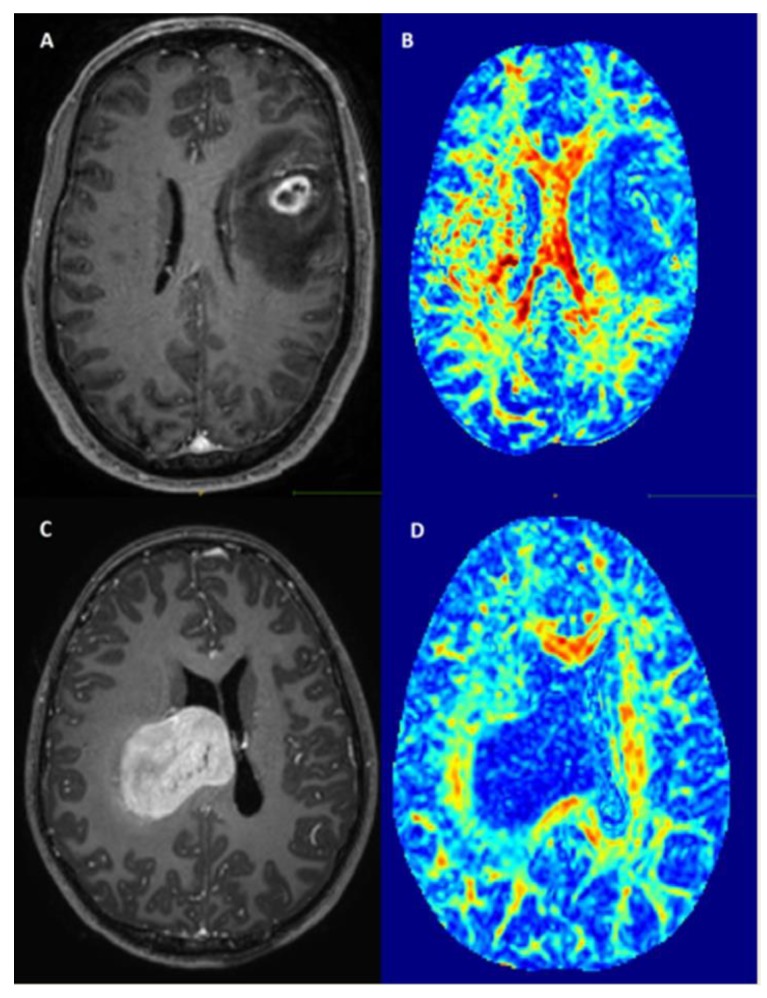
Examples of patients with (A) = T1-weighted imaging after contrast agent, (B) = Fractional anisotropy maps) and without (C,D) preoperative seizures.
